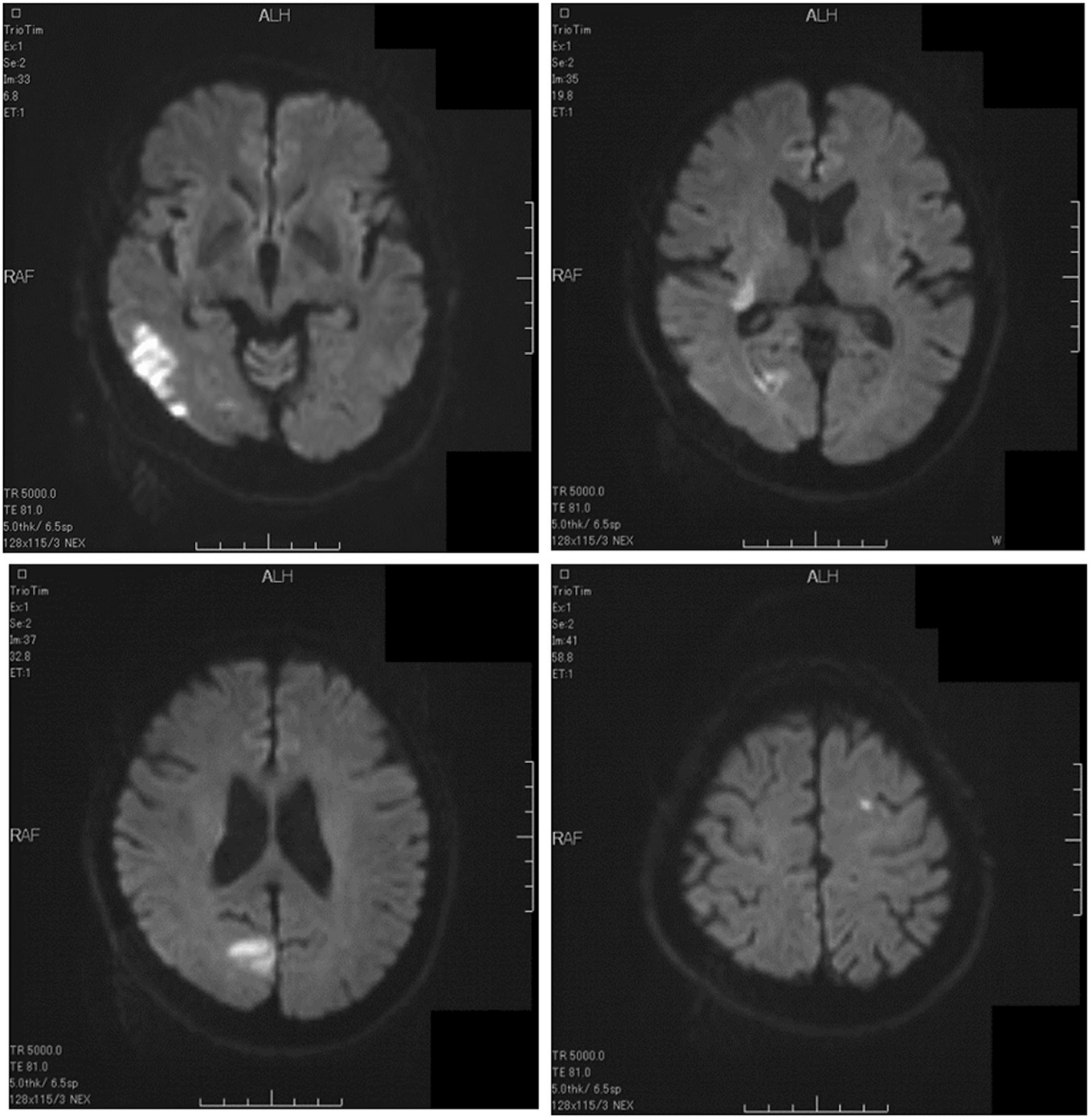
Brain magnetic resonance imaging scans show multiple cerebral infarctions.
